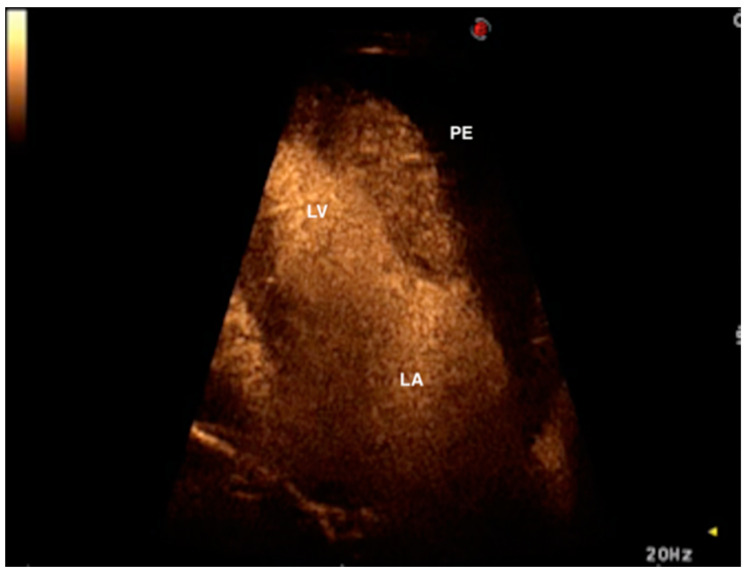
Contrast-enhanced echocardiographic image (left apical 4-chamber view) showing no evidence of microbubbles in the pericardial space after the complete opacification of the cardiac chambers. PE, pericardial effusion; LV, left ventricle; LA, left atrium.
